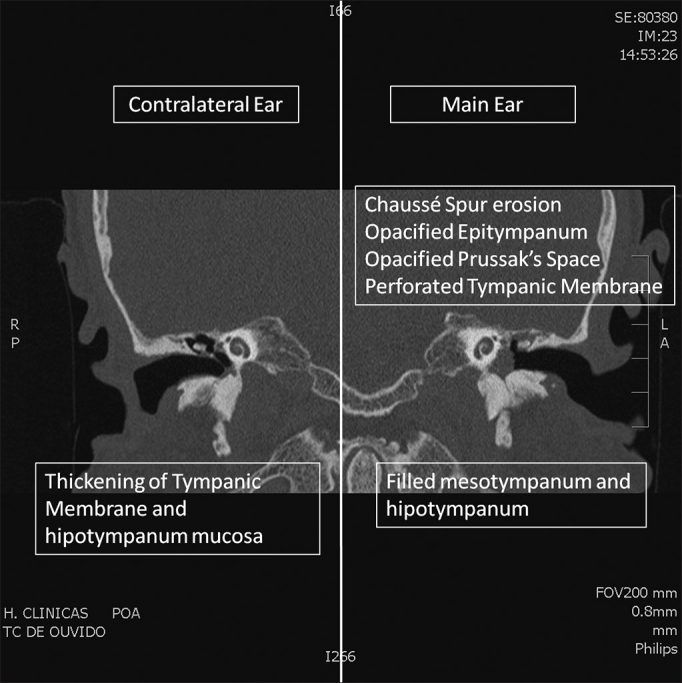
Coronal CT Scan. Comparison of abnormalities in the main (left) and contralateral (right) ear.
